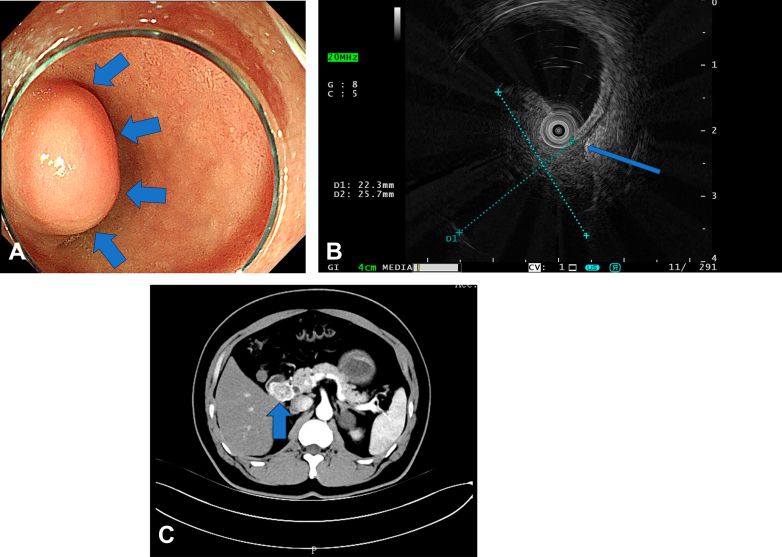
A, Endoscopy shows a mass in the duodenal bulb (*arrows*). B, Endoscopic ultrasound image showing 22.3 × 25.7-mm hypoechoic lesion arising from muscularis propria layer (*arrow*). C, Computed tomography image suggests a stromal tumor (*arrow*) in the duodenal bulb.
